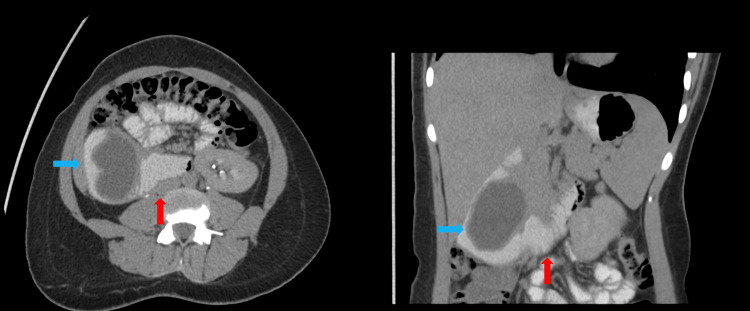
Axial (left) and coronal (right) right lateral decubitus images of CT abdomen with contrast showing an 8.3 × 4.0 cm tubular cystic lesion (blue arrows) in the second part of the duodenum extending into the proximal third of the duodenum (red arrows) The change in the shape of the tubular structure is evident across three phases: portovenous, post-oral contrast, and lateral decubitus (Figures 1-3). These findings suggest a large intraluminal DD (blue arrows) in the second and third parts of the duodenum, without signs of cholecystitis, diverticulitis, or other complications. DD, duodenal diverticulosis
